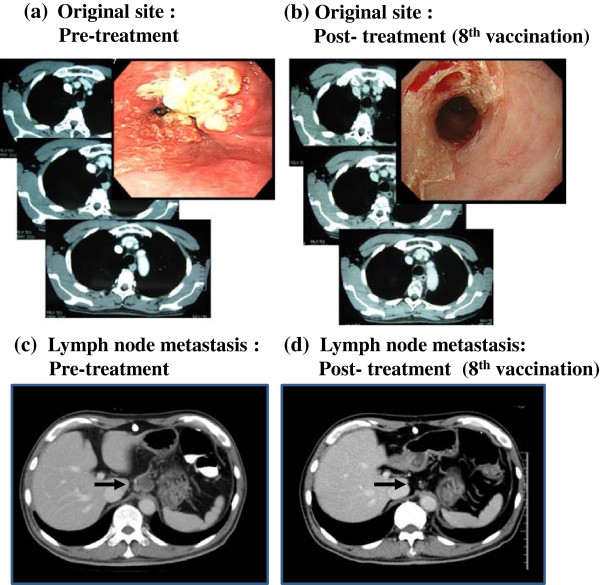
Computed tomography (CT) and endoscopic images of CR patient (case 6). The CT and endoscopic images of the original site (a, b) and CT images of lymph nodes metastasis (c, d) in case 6, before (a, c) and after treatment by CRT with 8^th^ vaccination (b, d) are shown.
